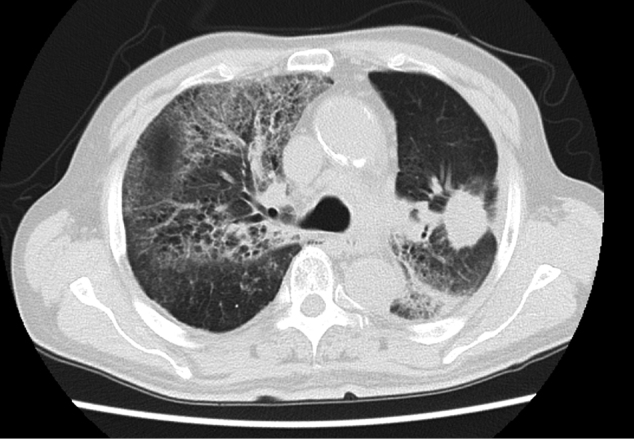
A lung CT image of a patient with locally advanced non-small cell lung cancer who had been treated with primary radiation therapy and later developed grade 4 radiation pneumonitis six months after completion of radiation therapy.
